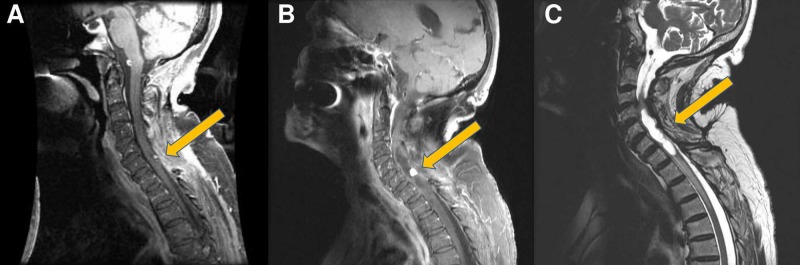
Recurrence of the hemangioblastoma and syrinx (A) Seven-month postoperative, fat-suppressed magnetic resonance imaging (MRI) with contrast shows a small enhancing lesion at C6; (B) four-year postoperative T1-fluid-attenuated inversion recovery (FLAIR) MRI with contrast; (C) MRI fast recovery fast spin echo (FRFSE) images show lesion growth with the development of a syrinx.
